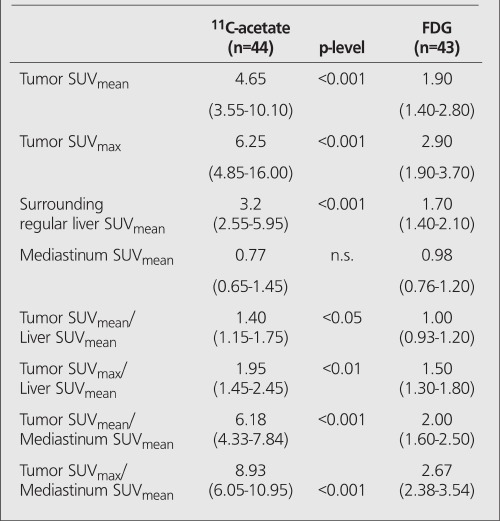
Median tracer uptake and inter-quartile range of the tumorat PET examinations using FDG and ^11^C-acetate in all patients withhepatocellular carcinoma. SUV’s and values vs. mean surroundingliver activity and vs. mean mediastinal blood activity, respectively, areshown
